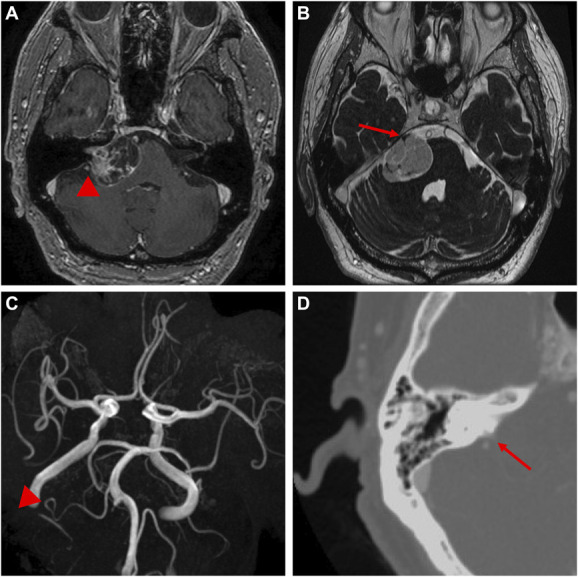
Preoperative magnetic resonance images. A, Gadolinium-enhanced T1-weighted imaging showed a right cerebellopontine angle tumor with enhancing solid and cystic components. A large vessel traverses the tumor dorsally (*arrowhead*). B, The trigeminal nerve was displaced anterosuperiorly on constructive interference in steady state imaging (*arrow*). C, Magnetic resonance angiography showed an aplastic right vertebral artery and PICA. A well-developed anteroinferior cerebellar artery PICA travels laterally and has an anomalous loop (*arrow*). D, Contrast-enhanced computed tomography showed a small dimple at the subarcuate fossa that contained a vessel (*arrowhead*). PICA, posteroinferior cerebellar artery.
